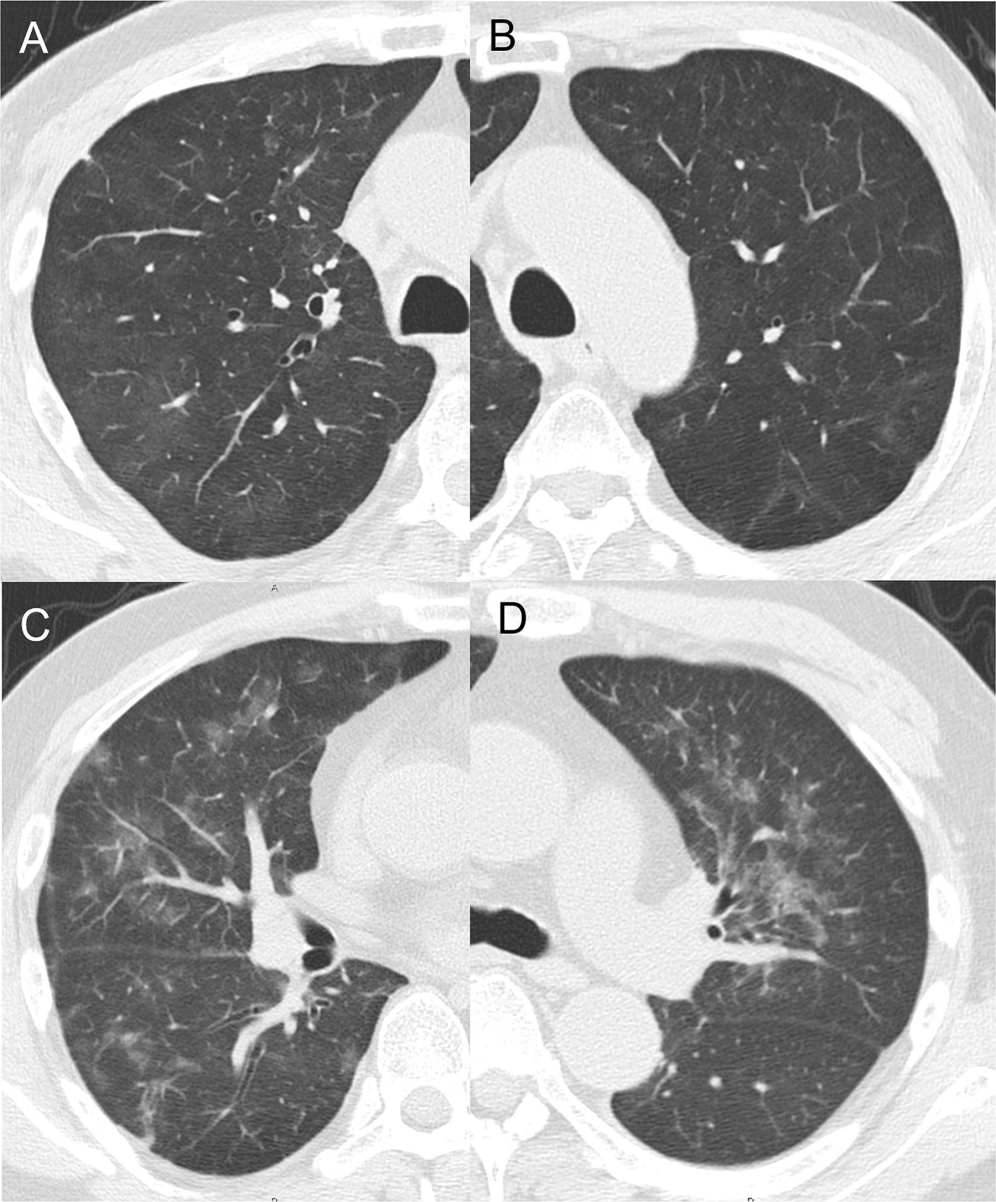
CT imaging of two patients with DI-IP. (A, B) A 67-year-old male patient with hypopharyngeal carcinoma after definitive CRT for primary disease with developing axillary lymph node metastasis. After PD with nivolumab, the patient underwent nine cycles of PC therapy. He was asymptomatic at the time of the CT scan, which was performed to assess the effectiveness of his treatment, and his KL-6 level was elevated to 550 U/ml (reference range: <500U/ml). However, later developed symptoms of dyspnea of the patient and was diagnosed as grade 2 DI-IP. The patient improved with follow-up. (C, D) A 57-year-old male patient with maxillary carcinoma after curative CRT for the primary disease developed localized recurrence where radical excision was impossible. After PD with nivolumab, he underwent nine cycles of PC therapy. He developed respiratory symptoms, such as fever and cough, and a CT scan to investigate the source of inflammation showed non-distracting frosted glass shadows in both lung fields. The serum KL-6 level was elevated to 860 U/ml. The patient was diagnosed as grade 3 DI-IP. He was treated with steroid pulse therapy and improved. CT, computerized tomography; DI-IP, drug-induced interstitial pneumonia; CRT, concurrent chemoradiotherapy; PD, progressive disease.
